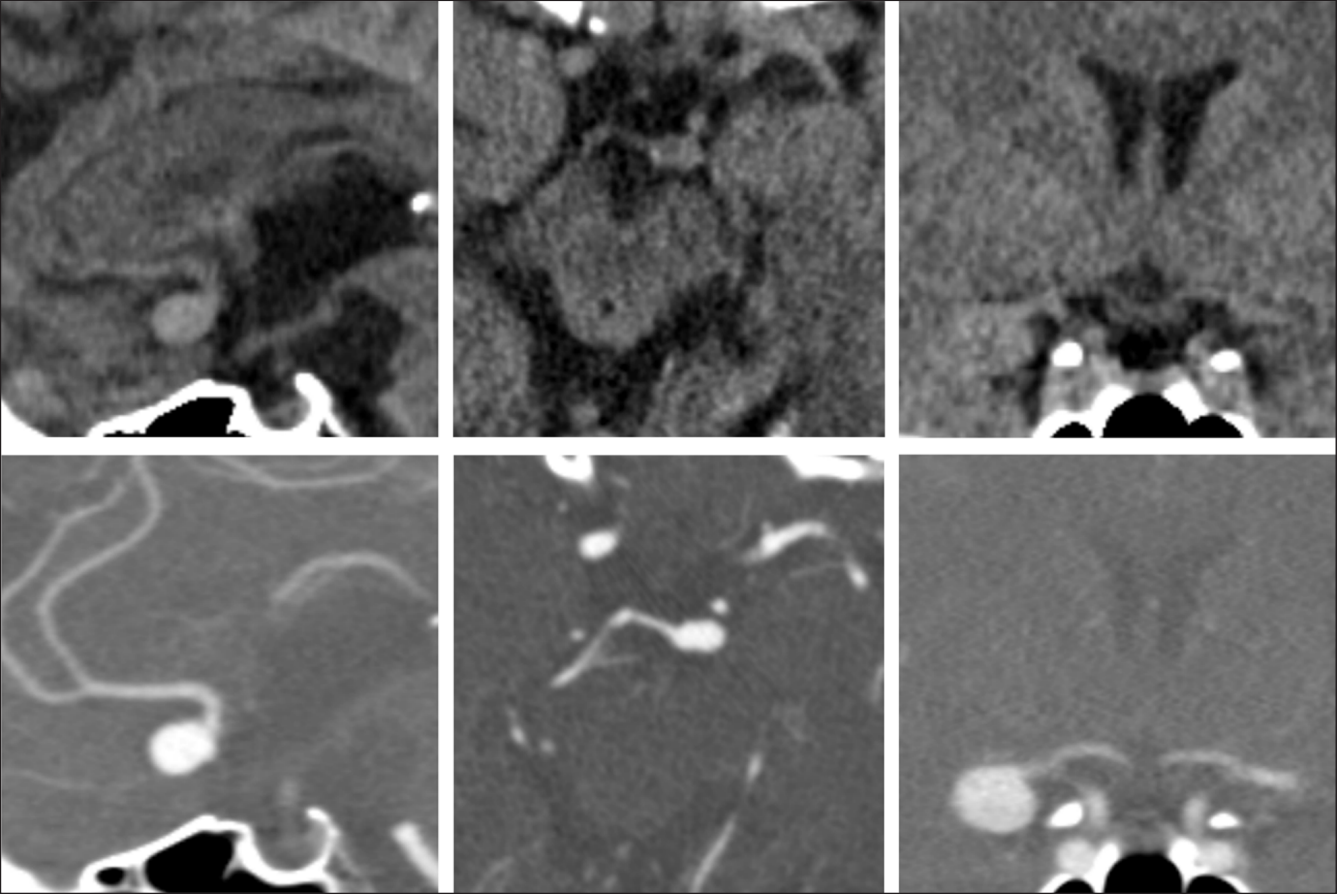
Examples of reconstructed images from the NECT datasets (upper row) and corresponding images from CT angiographies (lower row). Left: sagittal reconstruction of an aneurysm of the anterior communicating artery. Middle: axial reconstruction of an aneurysm of the basilar tip. Right: coronar reconstruction of an aneurysm of the middle cerebral artery.
